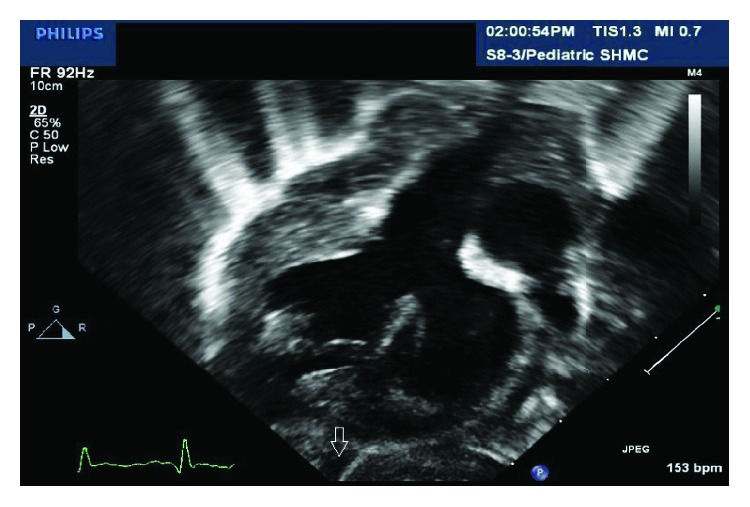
A preoperative transthoracic echocardiography demonstrating a left ventricular apical diverticulum (arrow) not noted at the time of examination.
